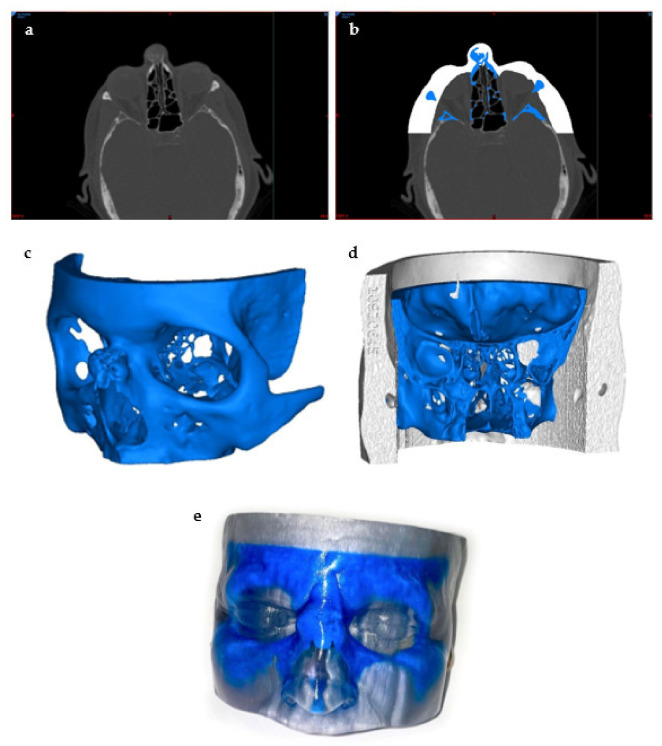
Preprocessing to 3D Printing of the 3D Model. This model was created to visualize a bone tumor in the nose: (a) visualization of the tumor using a CT scan of the head, (b) thresholding and segmentation to create separate masks of the skin and the tumor areas of interest using the segmentation software, (c) STL file creation of the bone tumor, (d) posterior view of the final STL model prior to printing, and (e) a photo of the final 3D-printed model.
